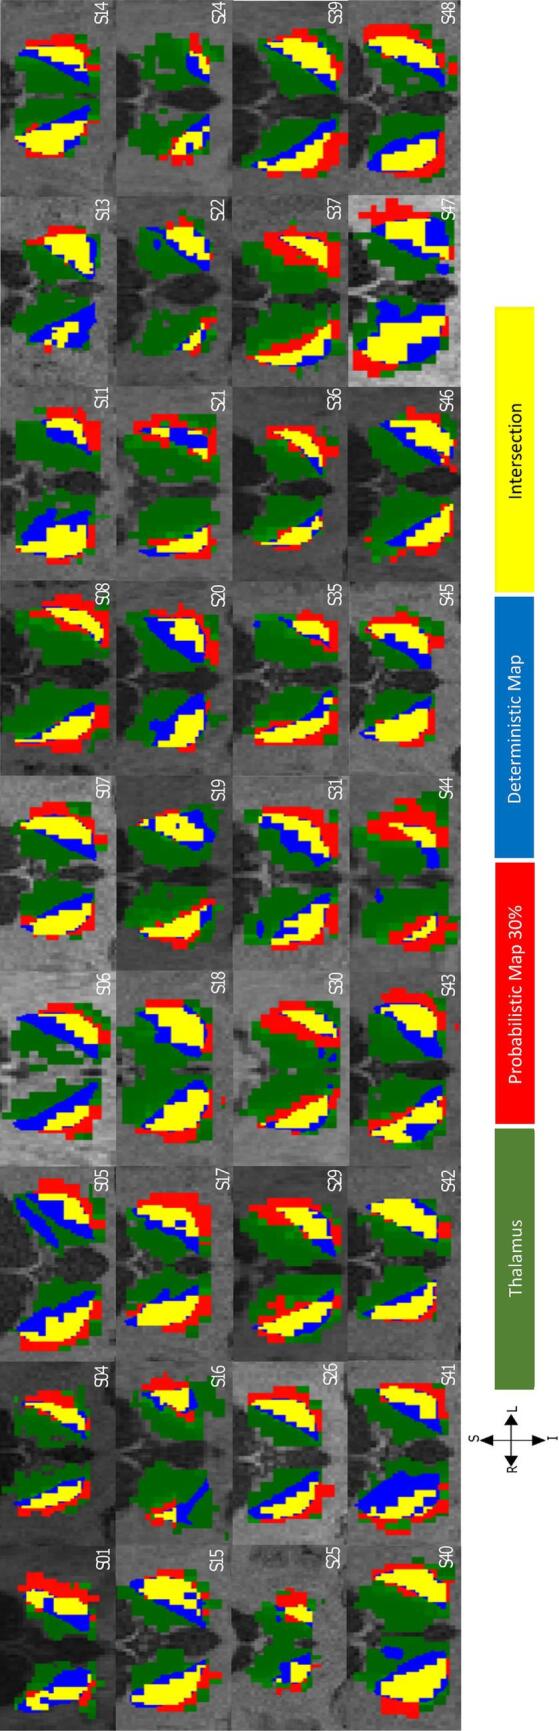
Qualitative comparison between deterministic and probabilistic thalamic segmentation maps. Coronal cross-sections of probabilistic (red) and deterministic (blue) thalamocortical segmentation maps and their intersection (yellow) in T1-weighted space for 36 patients with essential tremor. Both methods delineated the motor region in the posterolateral aspect of the thalamus (green). (For interpretation of the references to color in this figure legend, the reader is referred to the web version of this article.)
